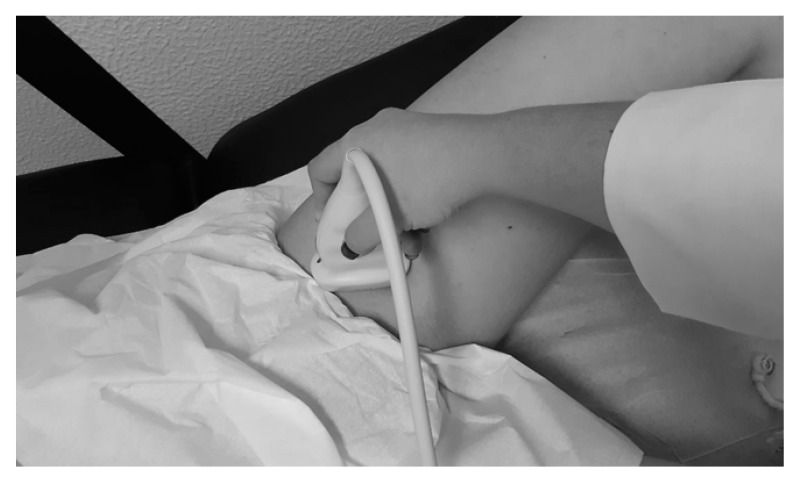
Transducer in position for doppler echo study in the proximal segment of the superficial femoral artery of the left lower limb.
